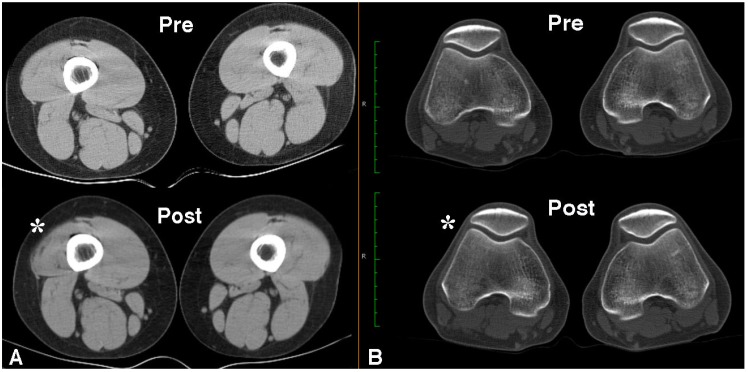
Axial CT images to highlight the anticipated focal atrophy seen in VL muscle at the 12 week follow-up review in the treated limb (*) following BoNT-A injection (A). Despite the induced atrophy, no change was recorded in force production nor congruency of the patellofemoral joint during quadriceps contraction at 30° knee flexion (B).
